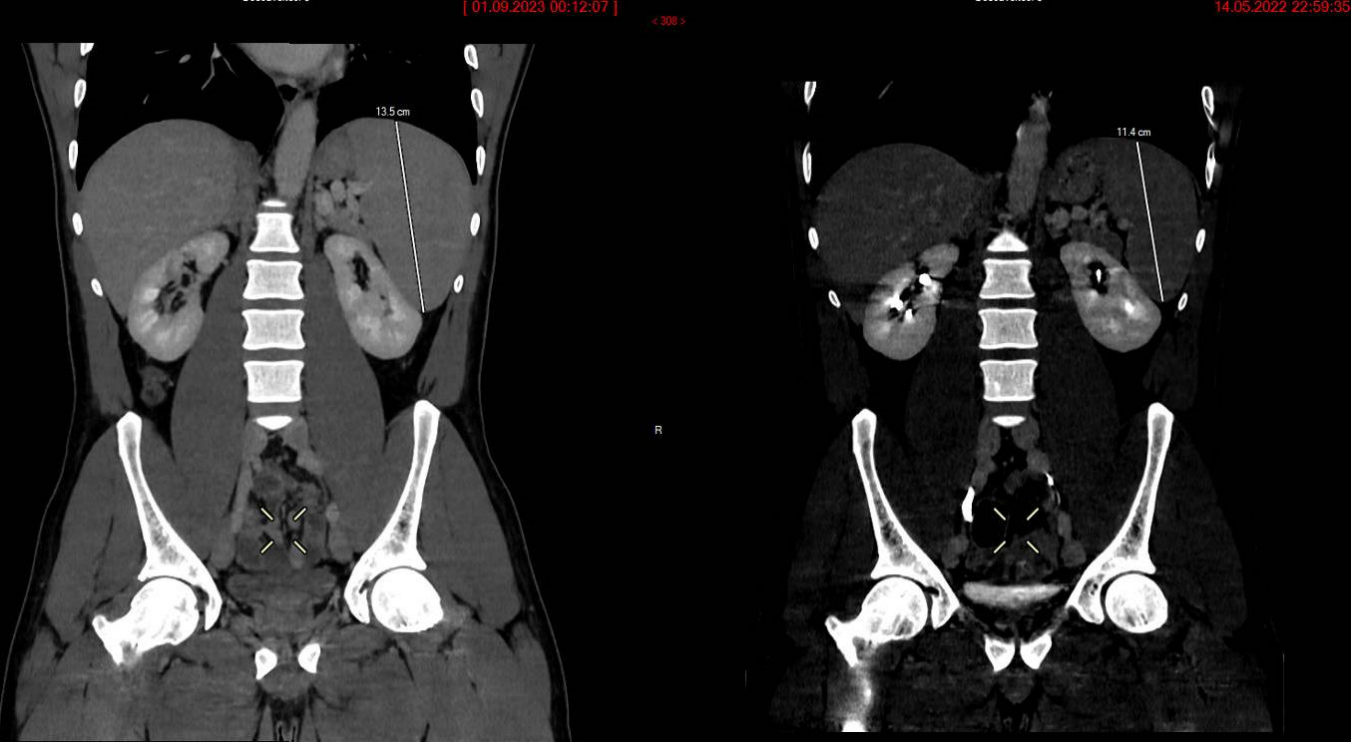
Abdominal CT scans showing worsening splenomegaly. The first scan (right) shows splenic dimensions of 11.4 × 12 × 5 cm. The second scan (left), 7 months later, shows increased spleen dimensions of 13.5 × 13 × 7 cm.
